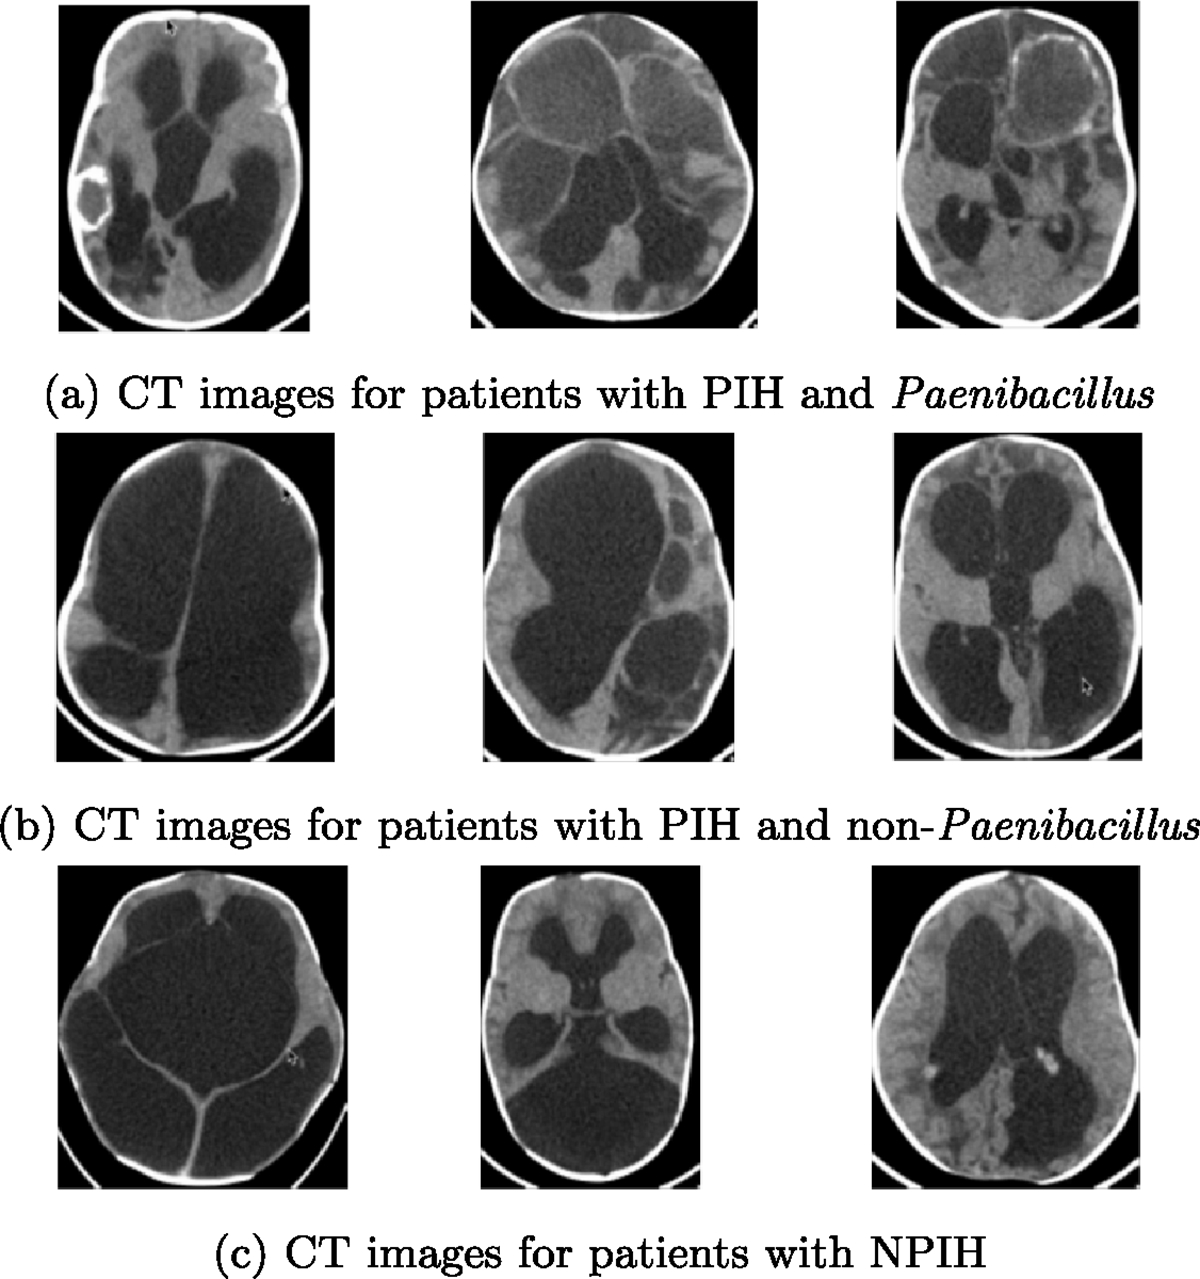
CT images from the CURE dataset.
